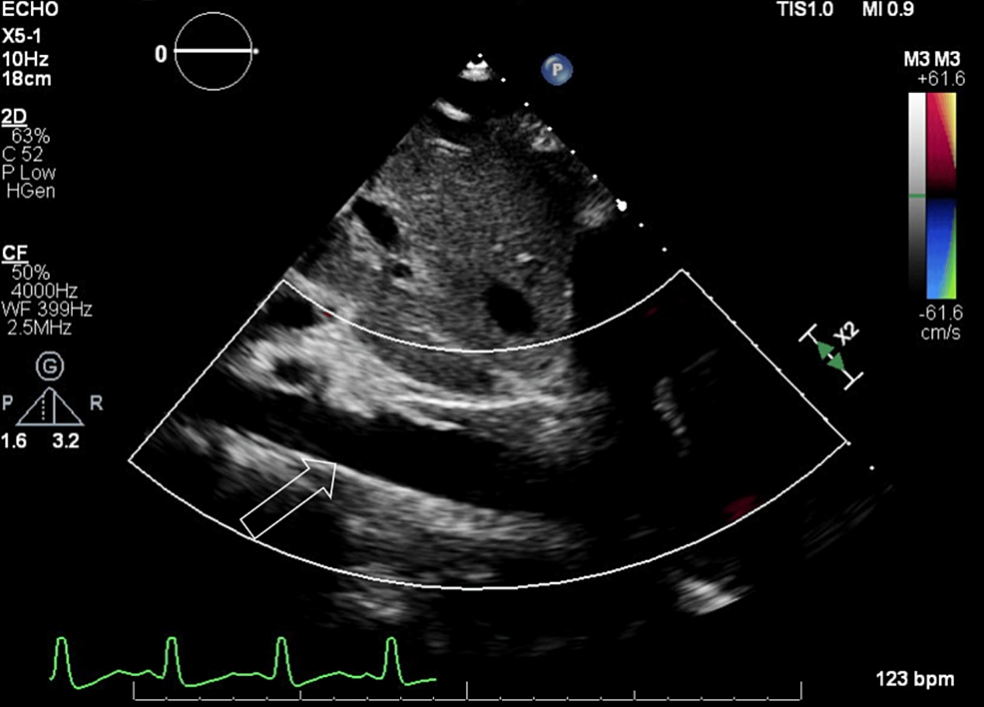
Dilated inferior vena cava visualized on echocardiography
